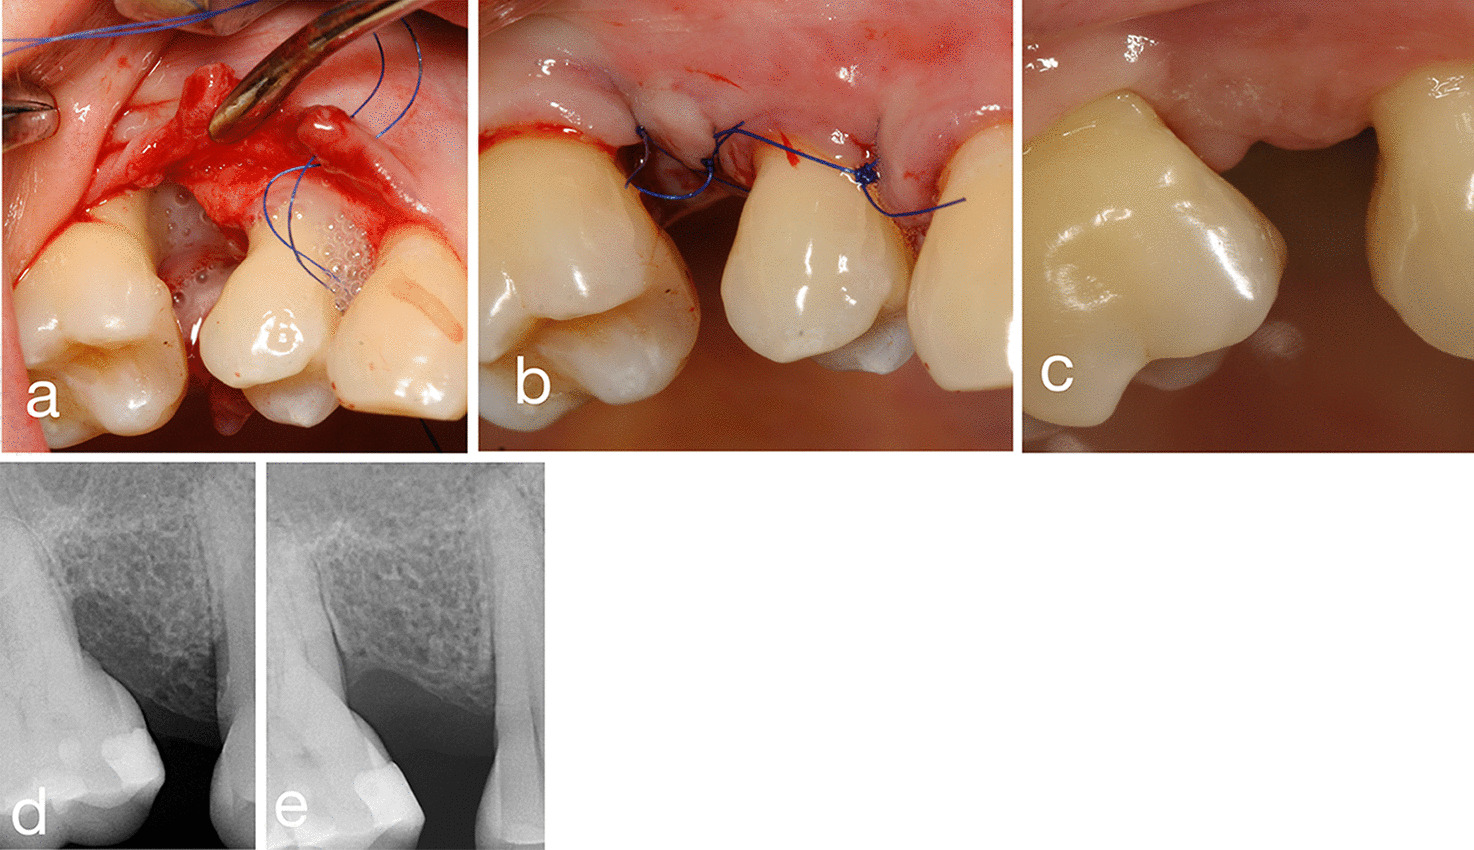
Treatment of an intrabony defect at an upper jaw molar with EMD. a Defect after debridement—intrabony defect filled with EMD, b Wound closure, c 6 months after surgery, d, e radiographic evaluation before and 6 months after surgery
